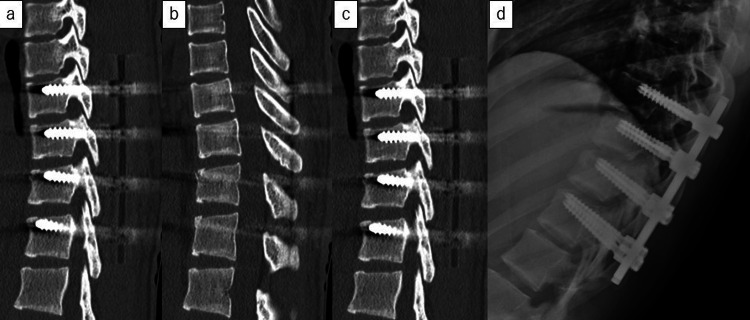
Postoperative imaging examination (a) Postoperative plain thoracic computed tomography (CT) of the left facet joint. (b) Postoperative plain thoracic CT in the sagittal plane. (c) Postoperative plain thoracic CT of the right facet joint. (d) Postoperative plain thoracic X-ray.
